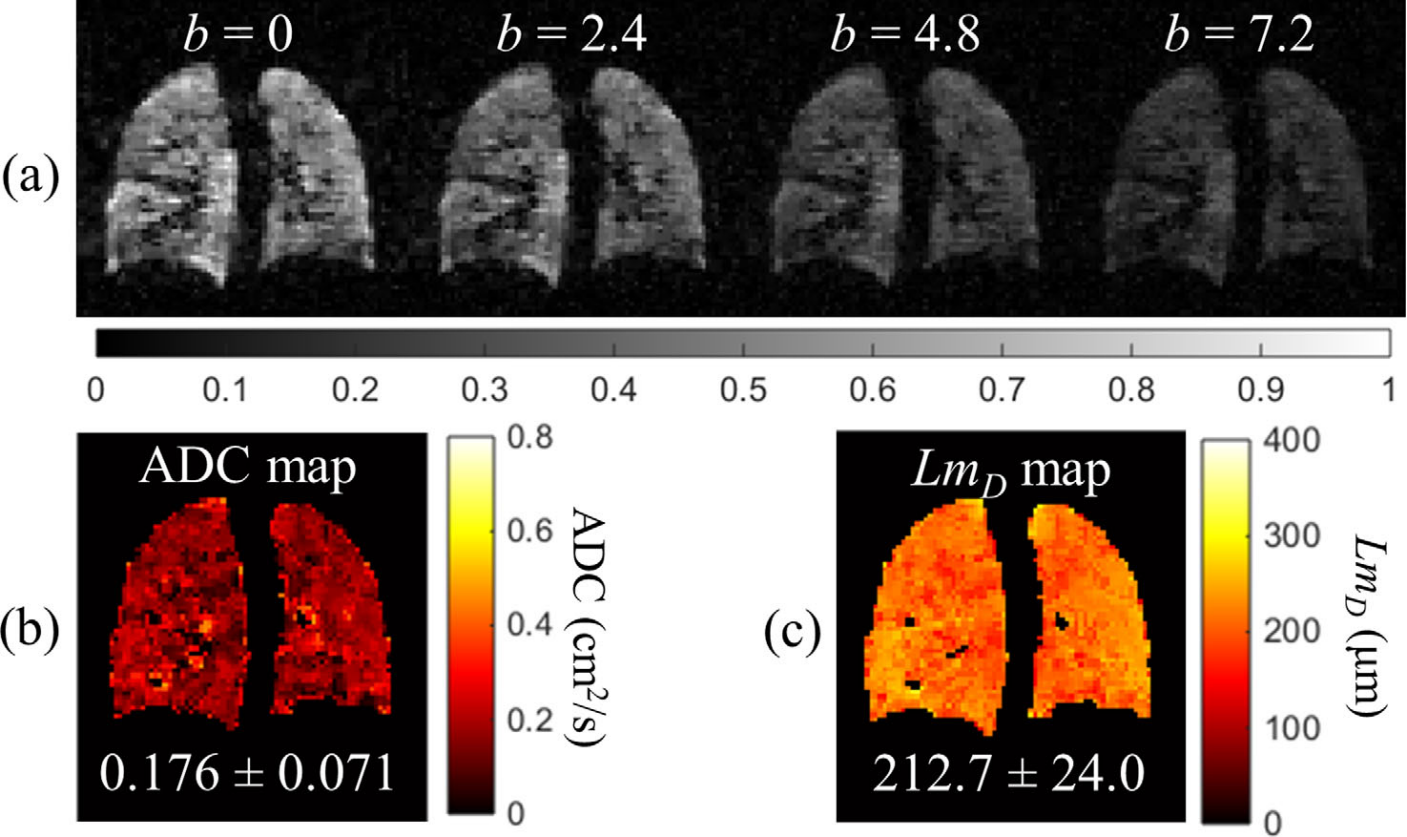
Prospective 3D ^3^He DW‐MRI CS acquisition from a healthy volunteer with three‐fold undersampling and four diffusion interleaves at *b*‐values of 0, 1.6, 4.2, and 7.2 s/cm^2^ (15 s breath‐hold). (a) Example slice for each diffusion interleave. (b) ADC map of the same slice calculated from the first (*b* = 0) and second (*b* = 1.6 s/cm^2^) interleaves. The representative mean slice ADC value is shown underneath the map. (c) Mean alveolar dimension (*Lm*
